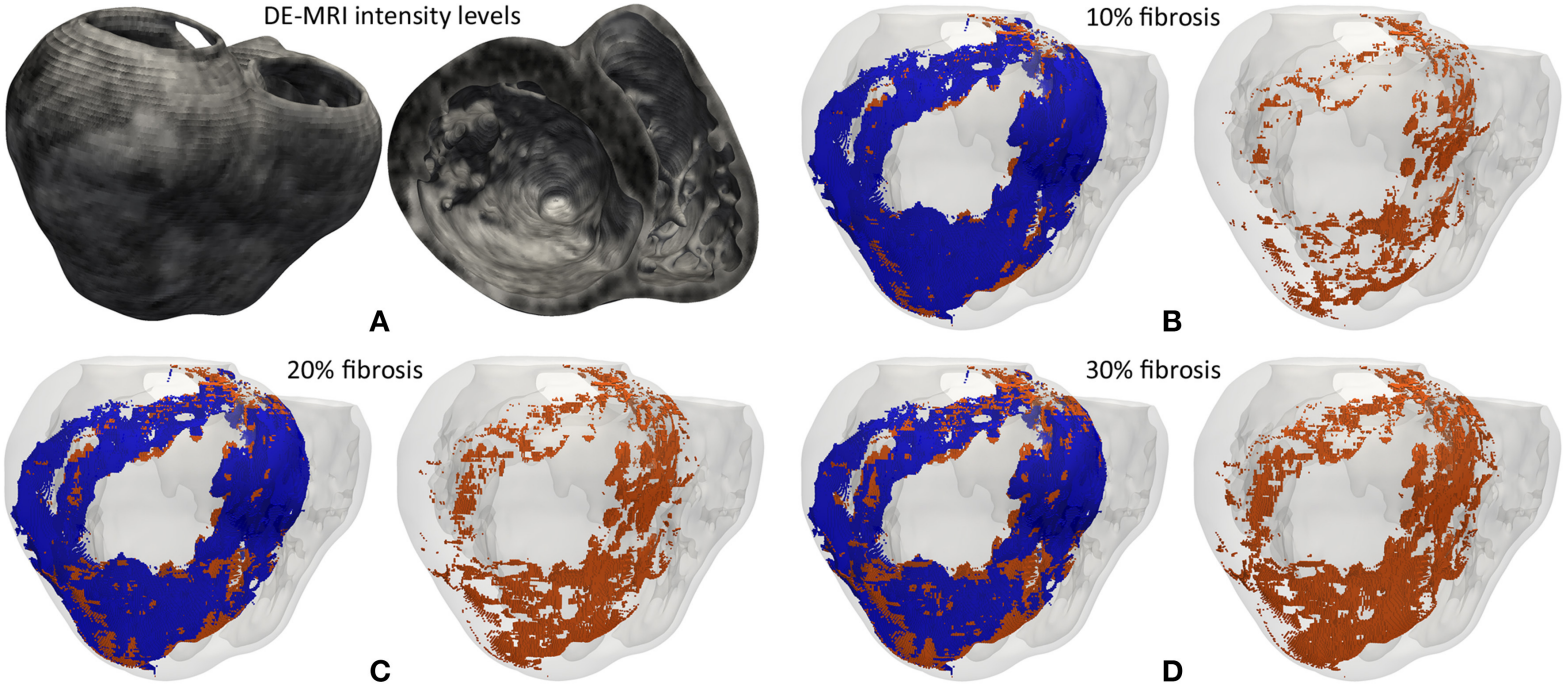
(A) Gray intensity levels from DE-MRI mapped into the hexahedral volume mesh of the 3D ventricular model, showing a postero-basal view of the whole model (*left*) and a basal view of a cross-section in the short-axis plane (*right*). The next three panels show the 3D fibrosis distribution resulting from the introduction of several degrees of structural remodeling within the BZ: (B) 10%, (C) 20%, and (D) 30% patchy fibrosis. All those panels show a posterior view of the 3D surface model of ventricles (rendered with transparency) together with those hexahedral elements labeled as BZ (*blue*) and fibrosis (*orange*) extracted from the volume mesh of ventricular model. In each panel there is an image showing both the BZ and fibrosis (*left*) and another one only displaying the fibrotic elements (*right*).
